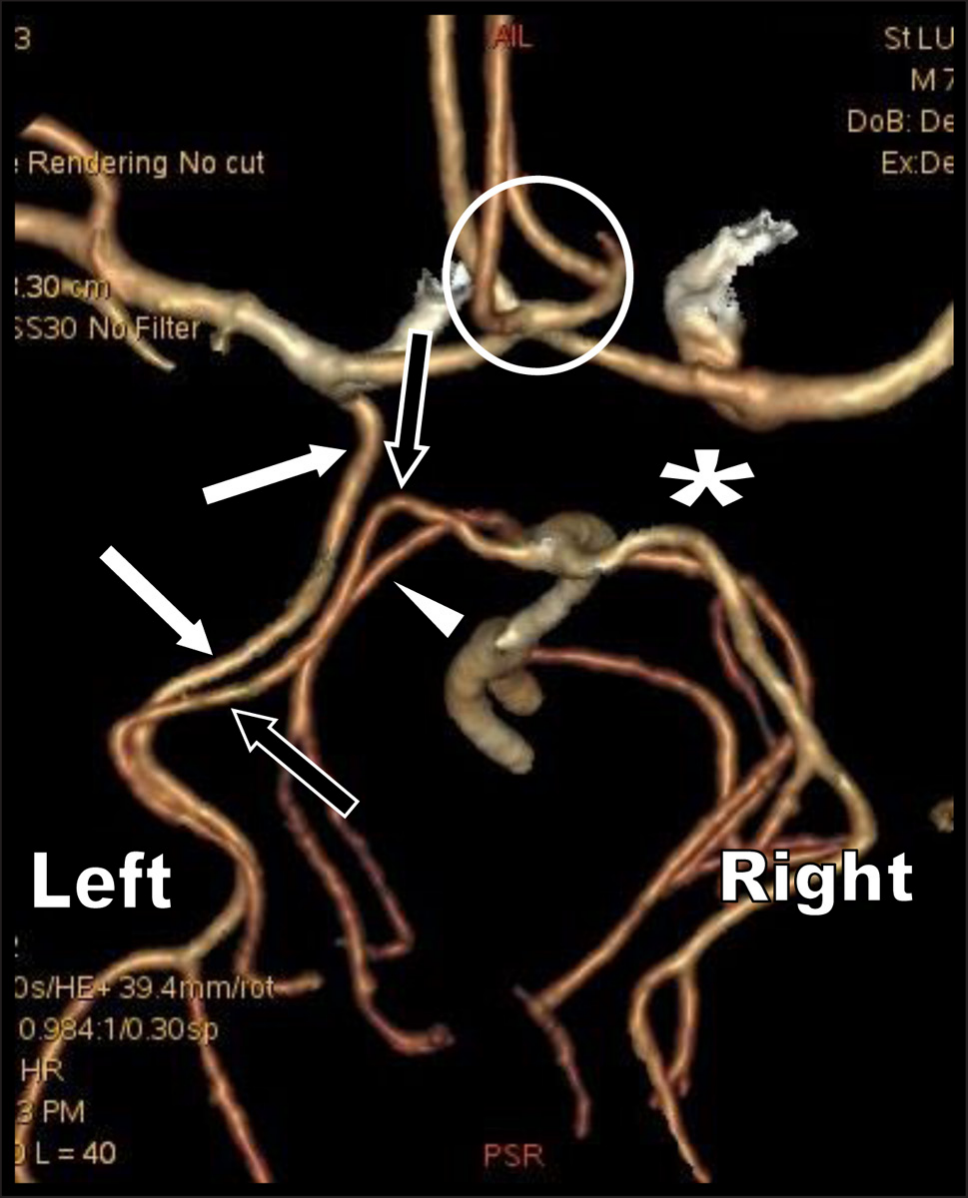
3D volume rendering view of the Circle of Willis obtained from the CT arterial phase. View from the vertex (left side is thus on the right of the figure).
